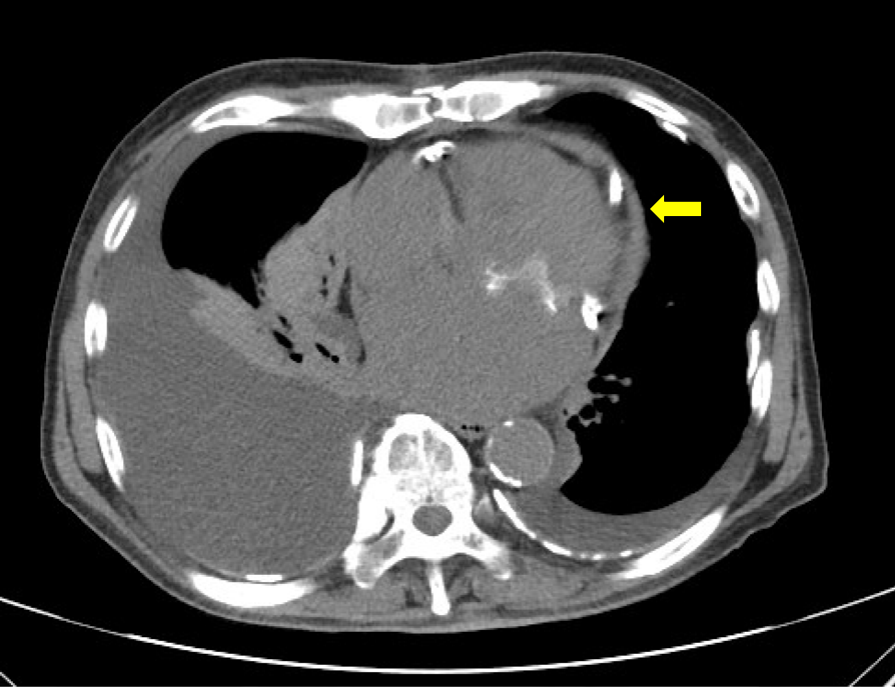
Computed tomography on readmission. There is a large amount of right pleural effusion. The pericardium is thickened and accompanied by calcification (arrow)
